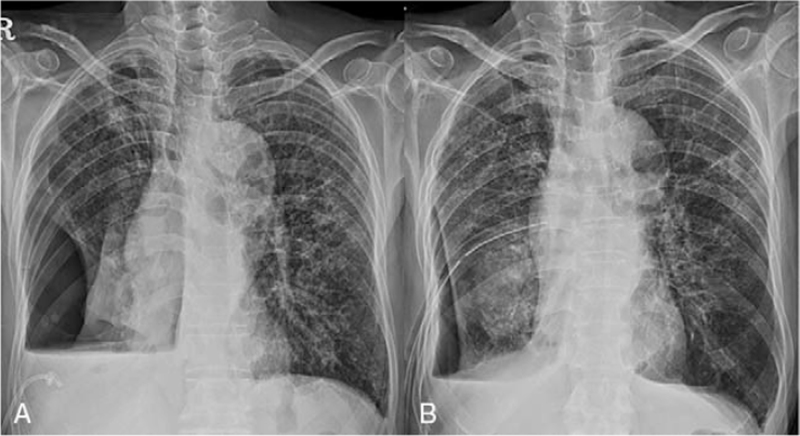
Chest radiographs showing (A) hydropneumothorax with pigtail catheter insertion in the right lung; and (B) hydropneumothorax with chest tube insertion in the right lung.
